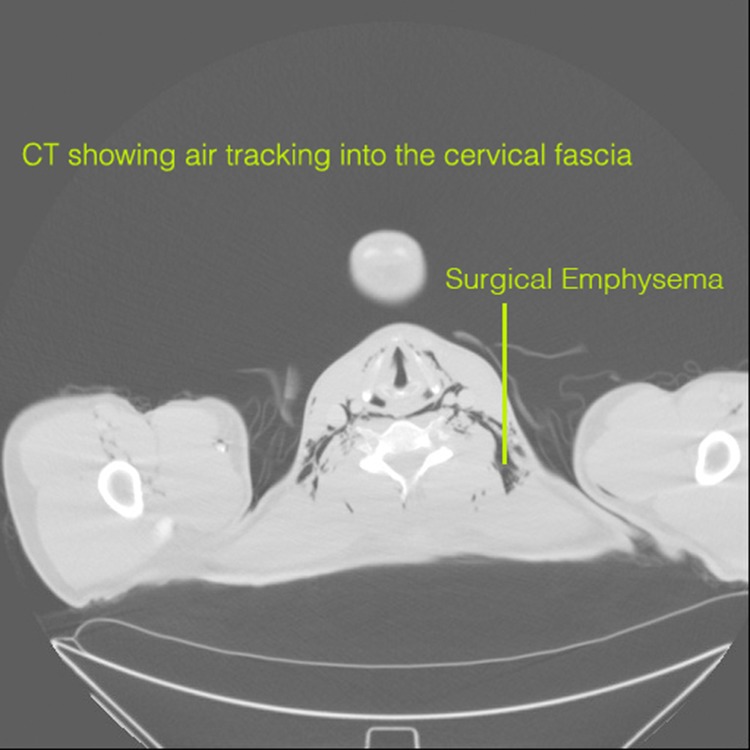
Computed tomography demonstrating subcutaneous emphysema in the cervical fascia
